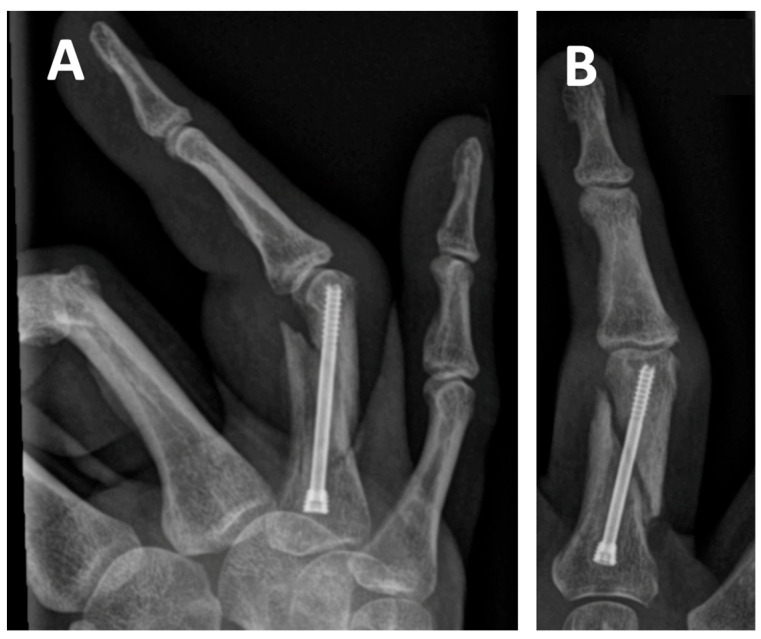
Radiographs of a 61-year-old female patient with a spiral fracture of the proximal phalanx of the right hand. The fracture was treated with antegrade intramedullary headless compression screw (IMHCS) fixation. Panels (A,B) show postoperative lateral and anteroposterior radiographs of the proximal phalangeal fracture. Due to inadequate fracture reduction, the patient developed mild clinodactyly as a residual deformity.
